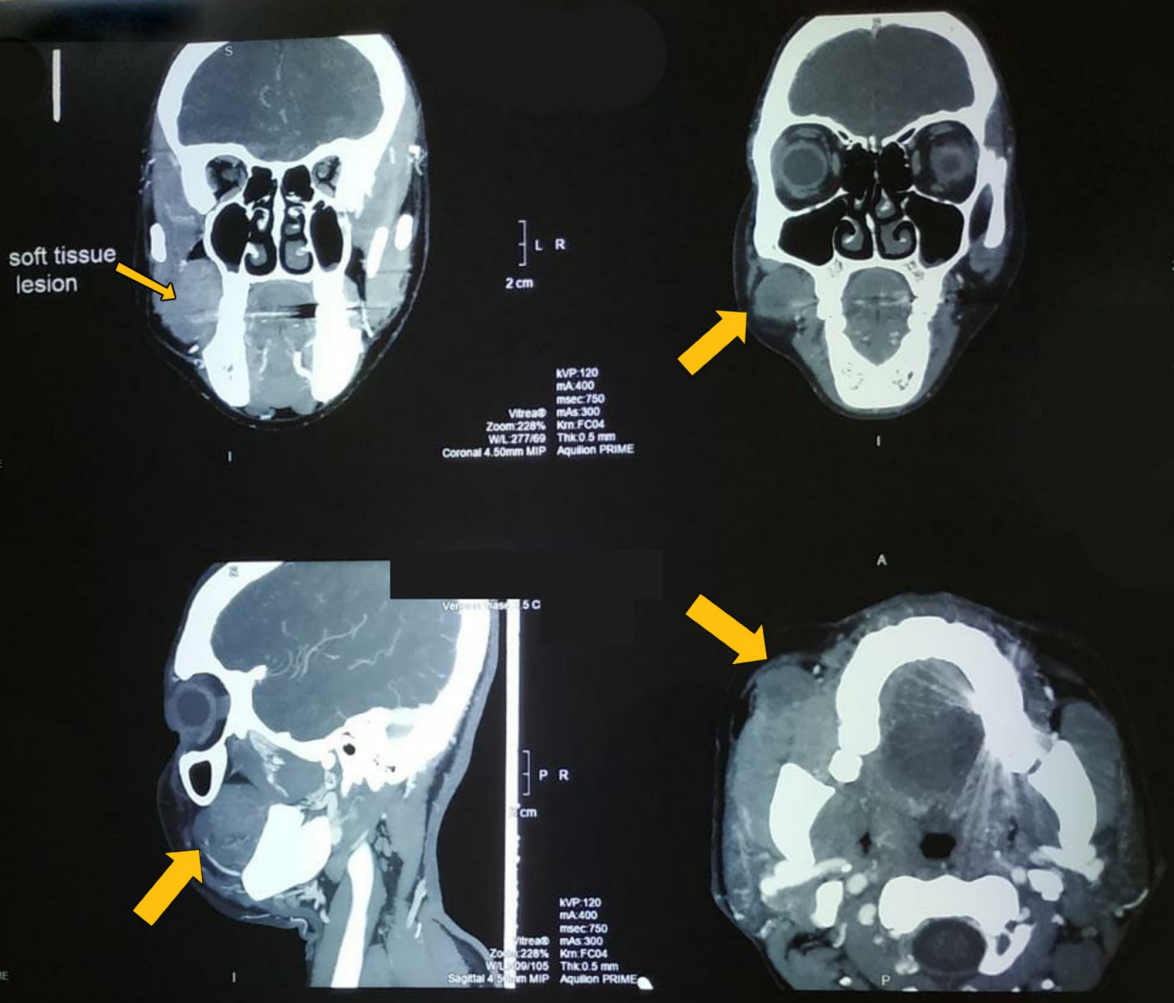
CT scan sections showing well-defined homogeneously enhancing lesion in the right buccal space region.
